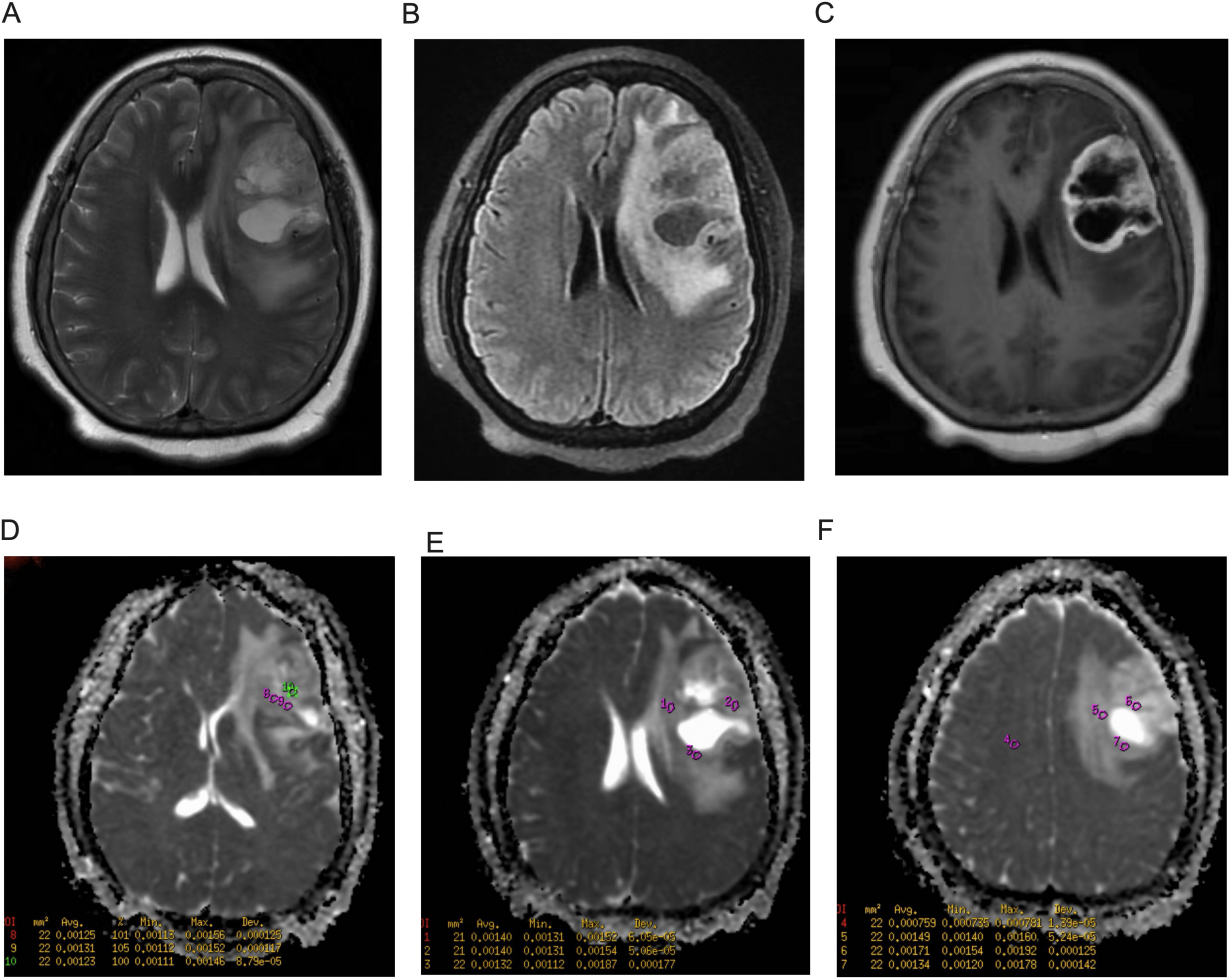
Region of Interest (ROI) Placement protocol for ADC quantification. (A) Axial T2-weighted image (T2WI) at maximal tumor cross-section. (B) Axial fluid-attenuated inversion recovery (FLAIR) image. (C) Axial contrast-enhanced T1-weighted image (T1WI-CE) delineating solid tumor enhancement. (D-F) Grayscale ADC maps with ROIs (color circles) at three tumor levels: (D) Slice with 3 ROIs; (E) Maximal cross-sectional slice with 3 ROIs (ADCmean = 1.37 × 10^-^³ mm²/s); (F) Slice with 3 ROIs + contralateral reference ROI (ROI 4). ADCmin (1.11 × 10^-^³ mm²/s) derived from the lowest value among all 9 tumor ROIs. All images cropped to remove peripheral space. Patient: 73M, IDH-wt glioblastoma.
